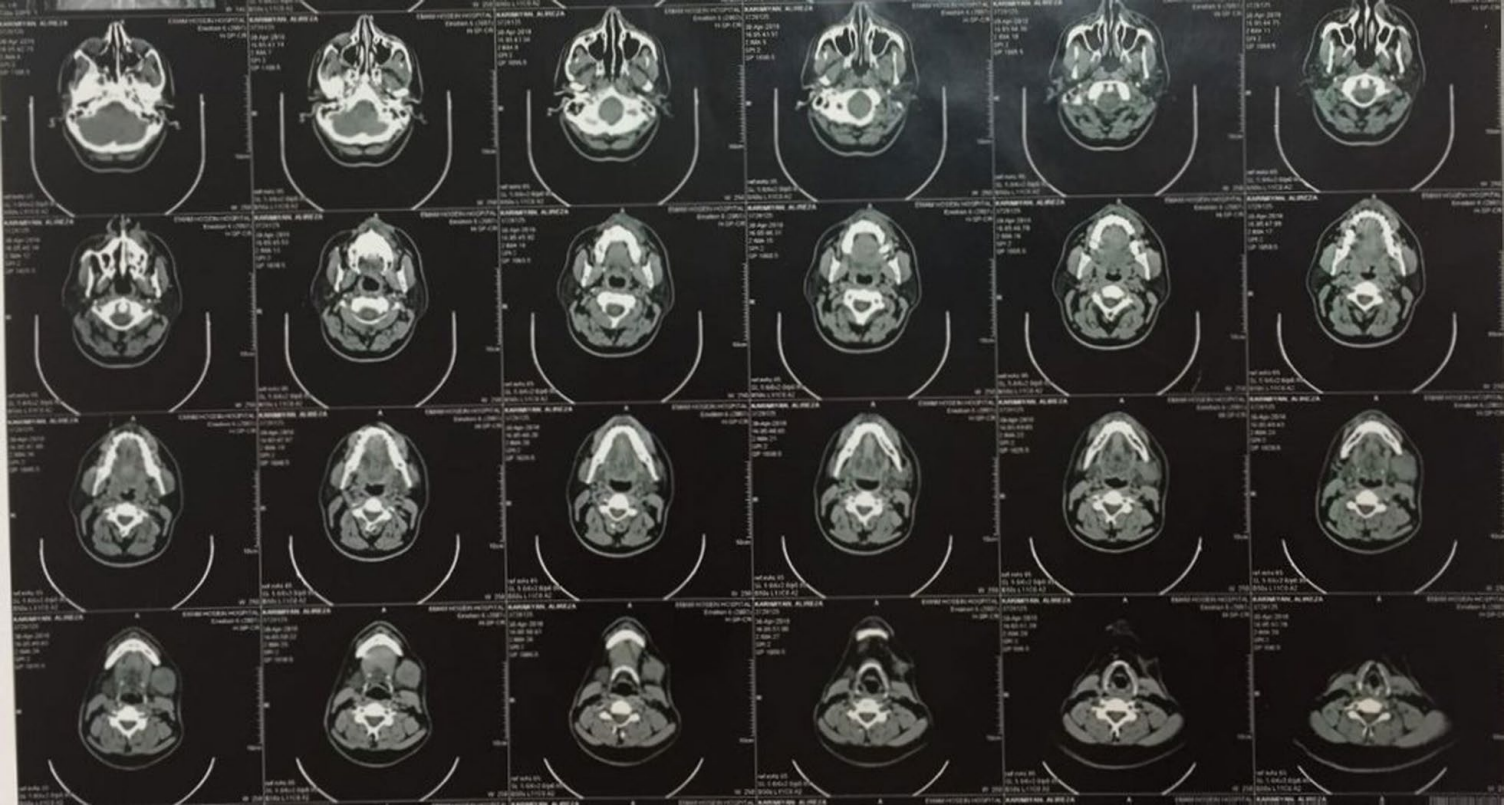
Cone beam computed tomography (CBCT) demonstrating soft tissue mass in the left submandibular gland and several oval shape cervical lymph nodes
